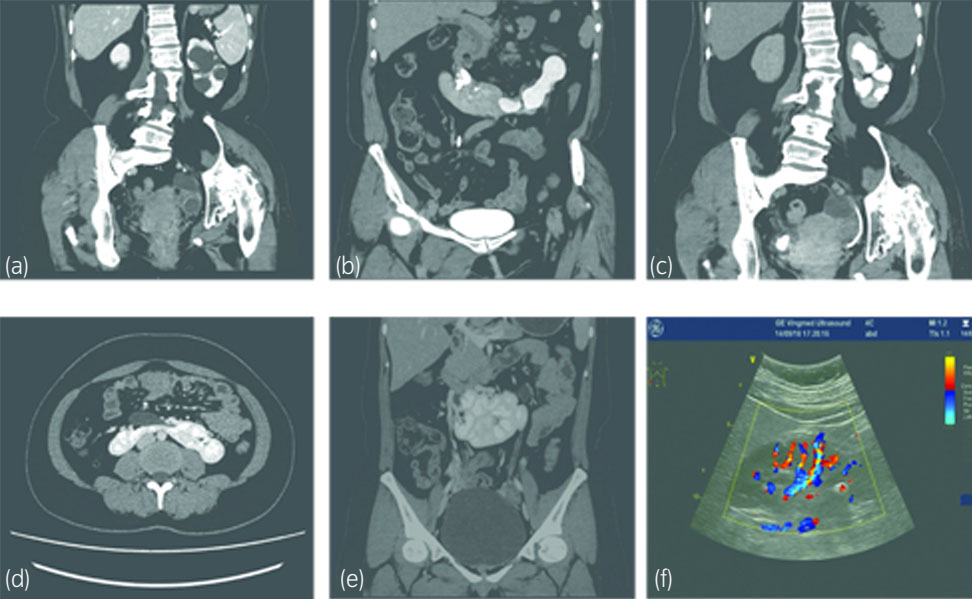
Preoperative CT scan of the horseshoe kidney and postoperative follow‐up image (Case no. 3, a, b: preoperative CTU, c: postoperative CTU at 8 weeks; Case no. 5, d, e: preoperative CTU, f: ultrasound image at 8 weeks).
